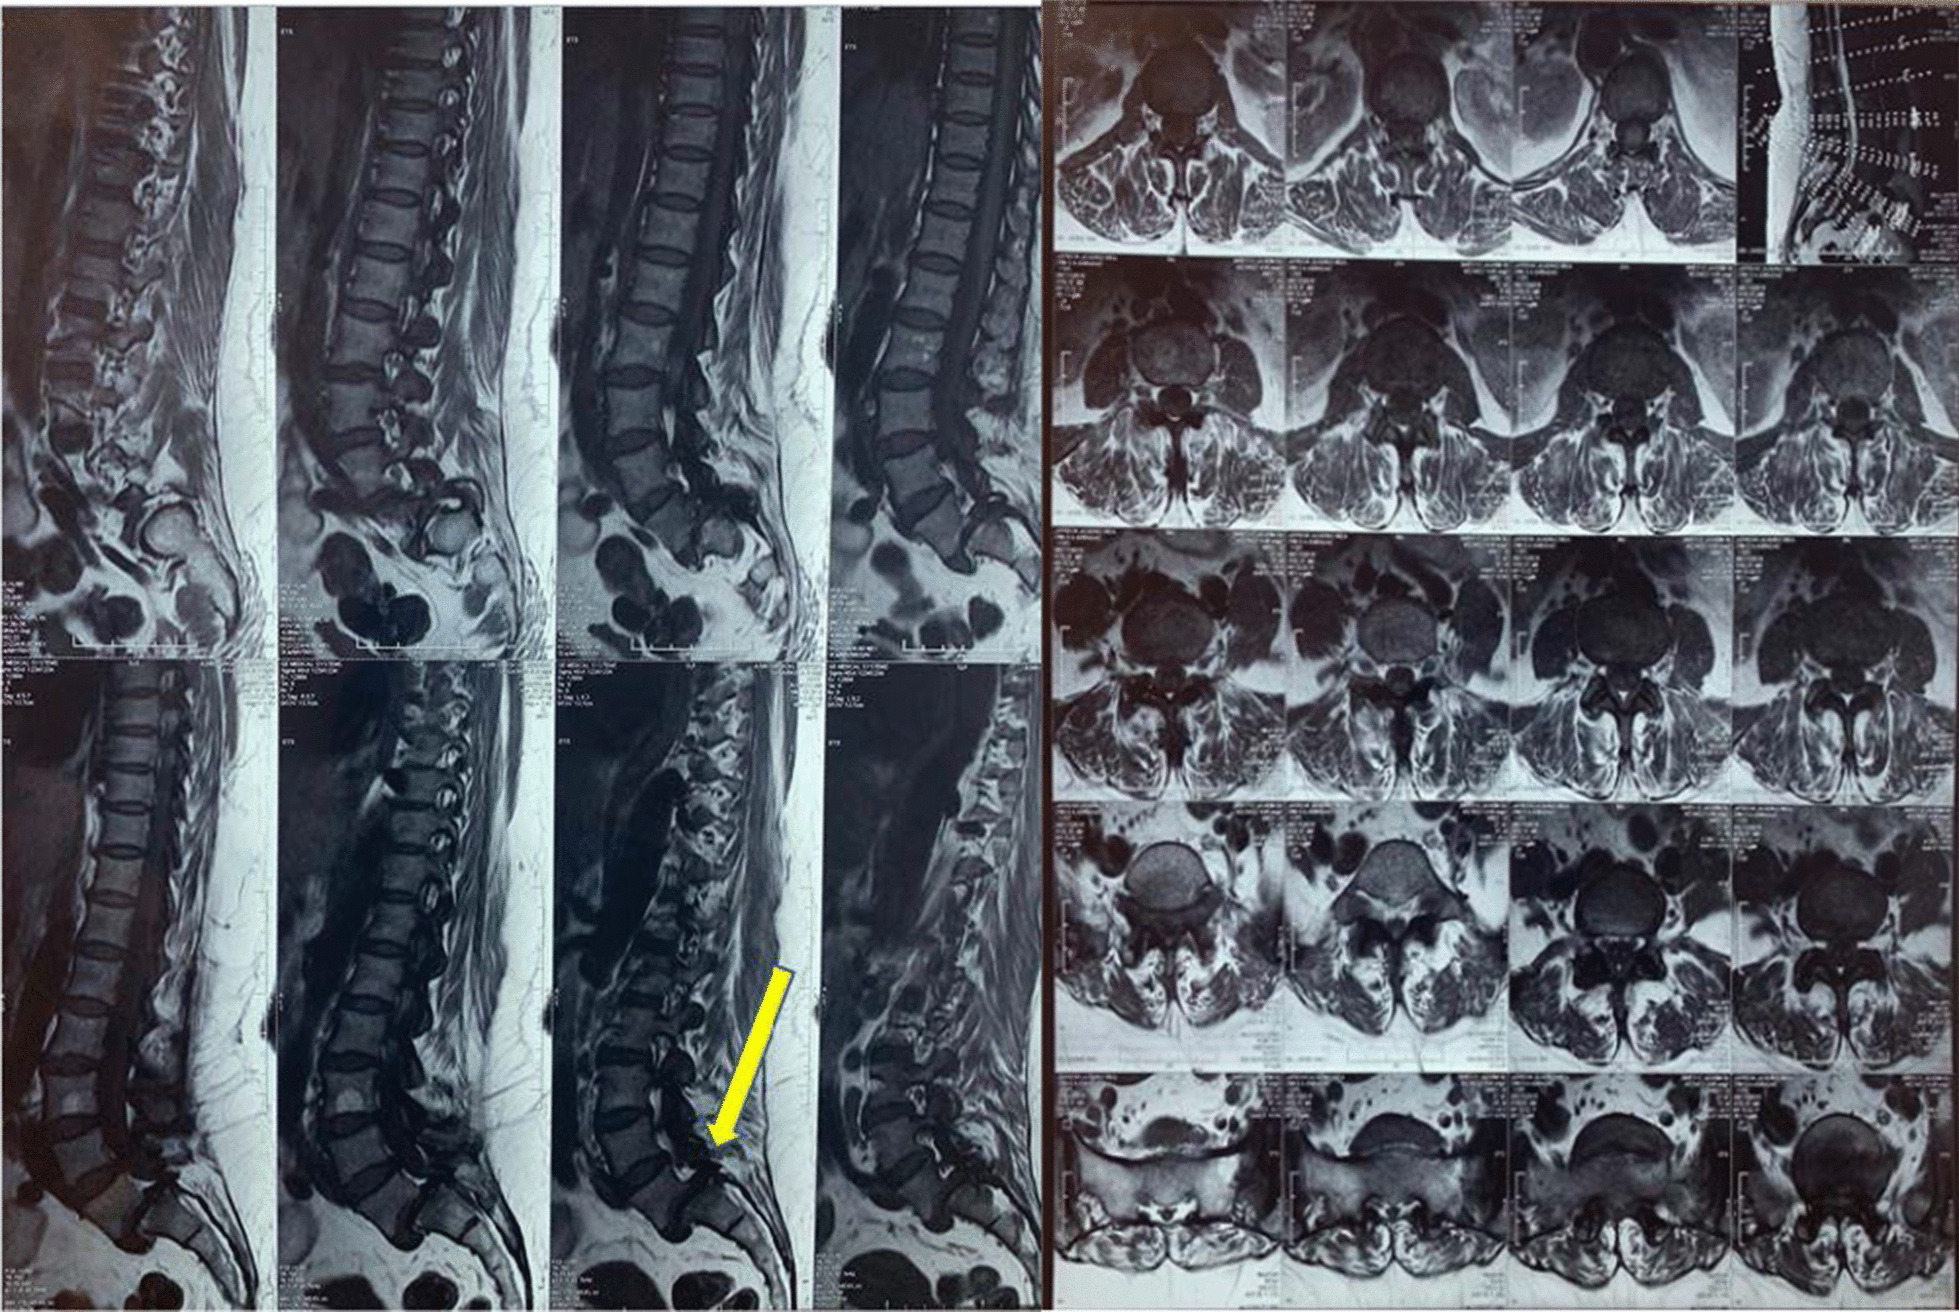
Sagittal and axial lumbosacral MRI showing L5–S1 spondylolisthesis. Arrow shows the site of spinal cord compression
